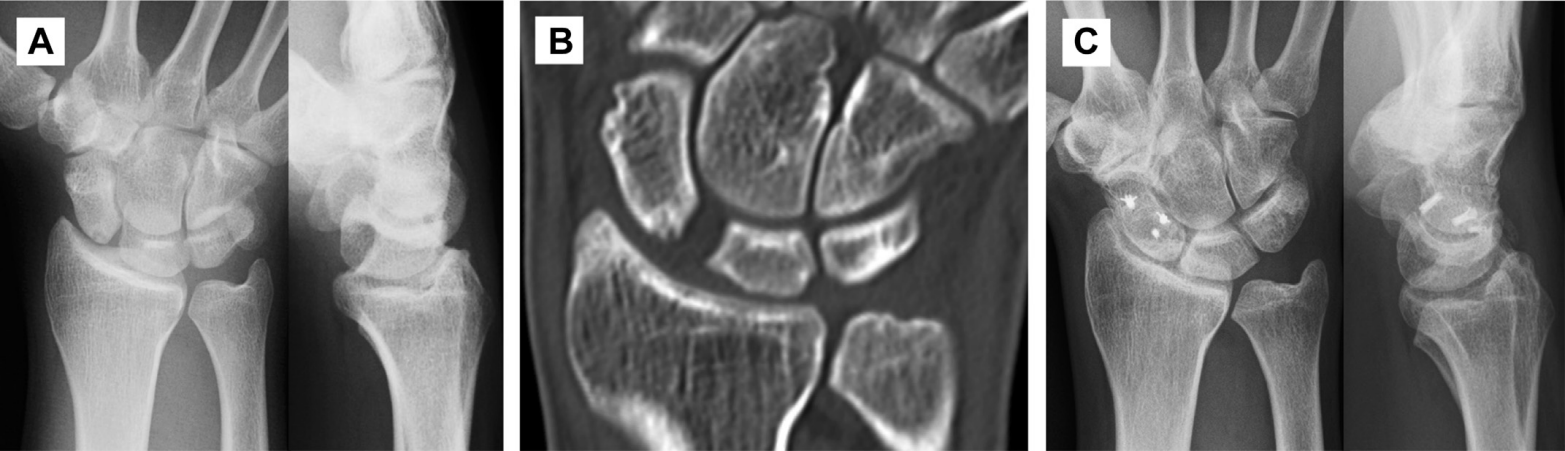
Radiological findings of patient 1. A and B Preoperative radiographs and computed tomographic imaging showing scapholunate instability (SLG: 4.2 mm; SLA: 97.2°). C Radiographs at 9.5 years after surgery showing normal carpal alignment (SLG: 1.6 mm;SLA: 60.7°). Osteoarthritic changes such as a slight narrowing of the joint space and sclerosis of the subchondral bone at the radioscaphoid joint were observed.
